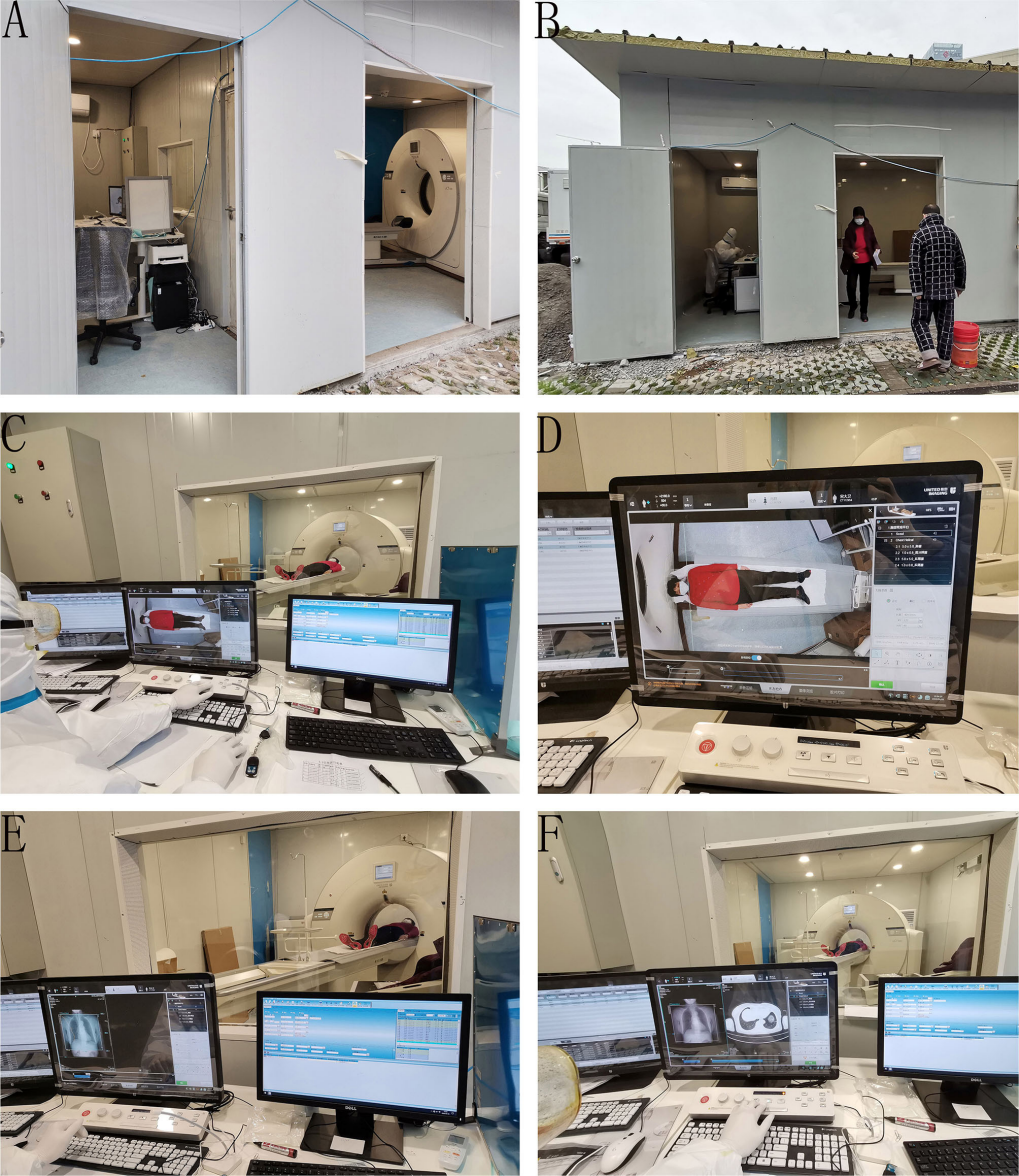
Operation process of relocatable CT aided by automatic positioning technology. (A) The inner structure of the relocatable CT system. (B) The patients enter the scanning room from a separate door by themselves. (C) The examination bed moves to the ideal position automatically with the help of the camera-based intelligent auxiliary positioning system. (D) The patient's real-time image is transmitted to a computer screen in the controlling room. (E) The automatic positioning system determines the scan range automatically. (F) The technologist starts scanning.
